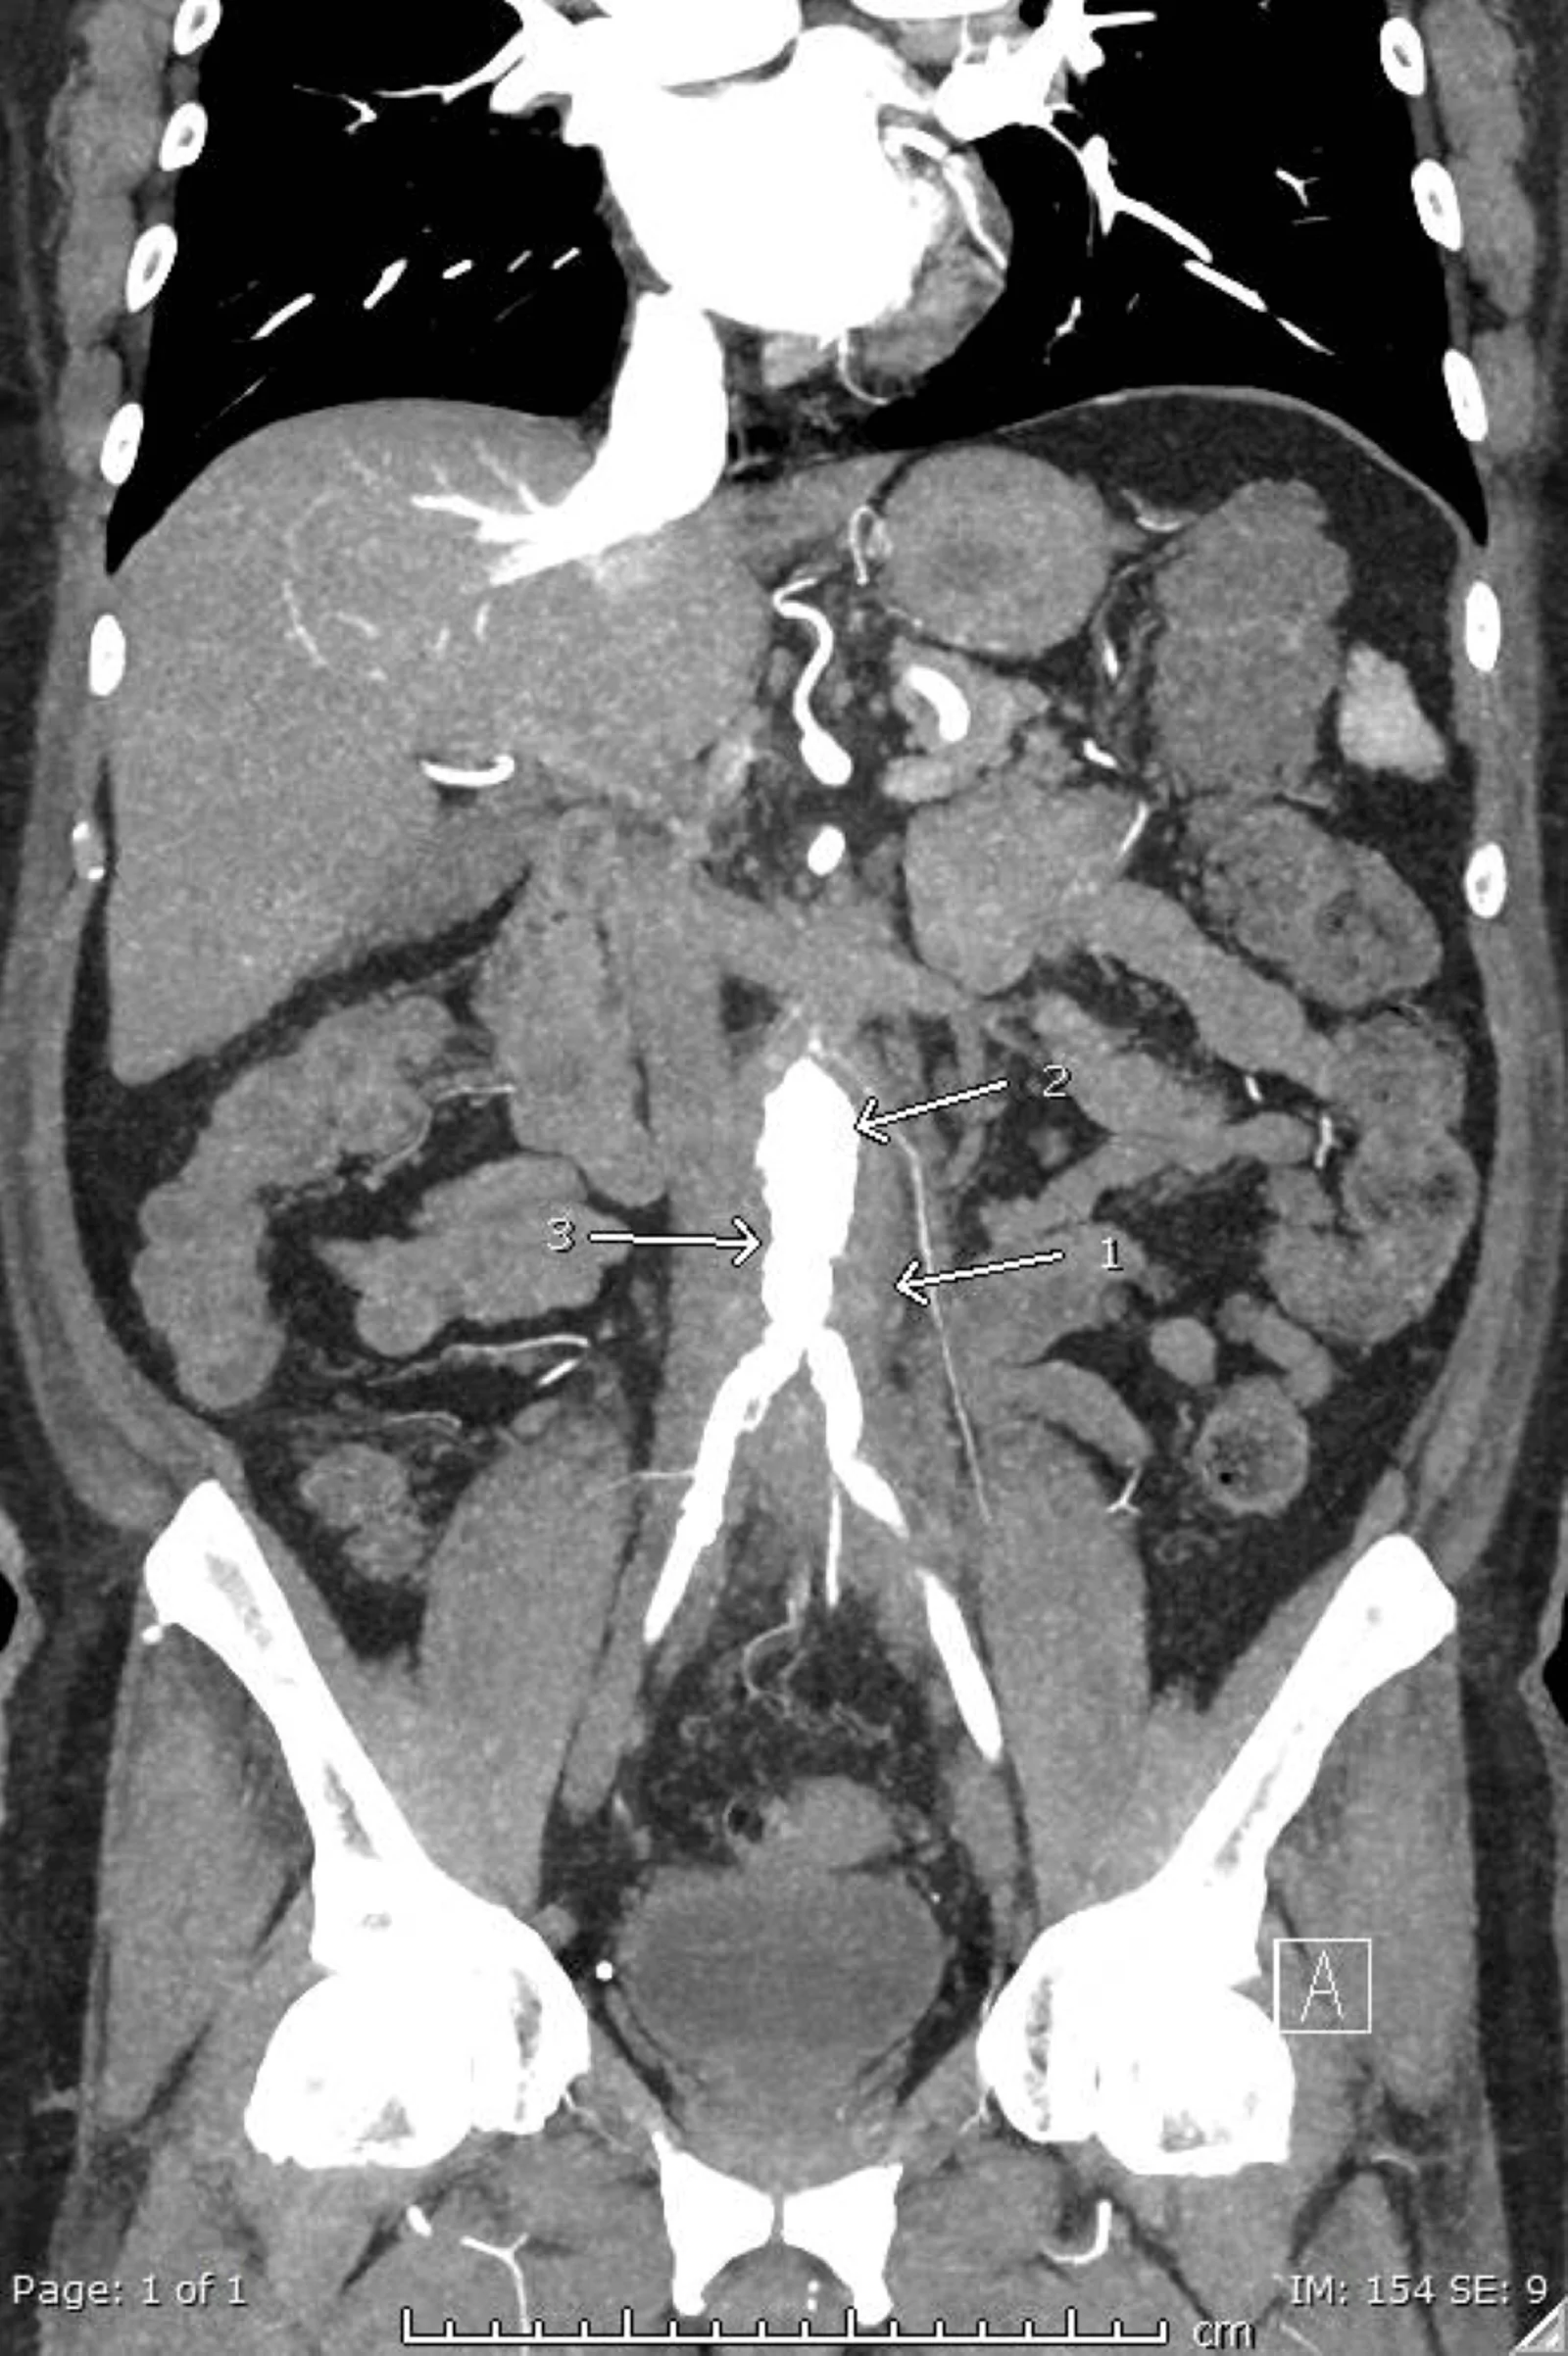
CT angiography of the abdomen (sagittal plane) showing periaortic inflammation and retroperitoneal fibrosis (arrows)
